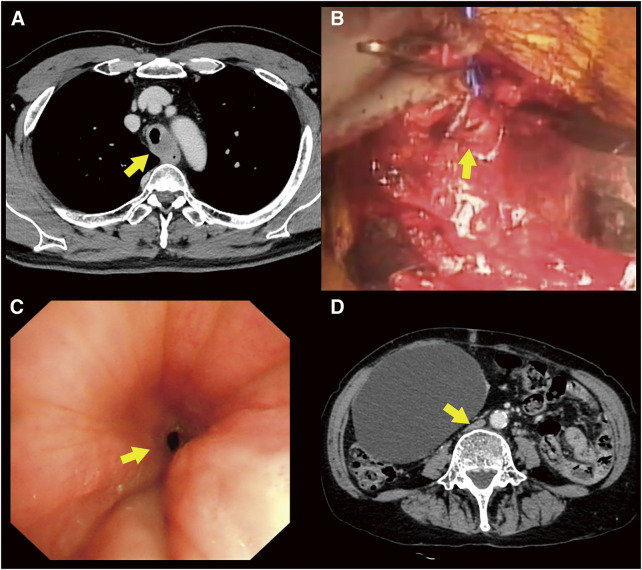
(A) Contrast-enhanced CT revealed a tumor 4.7 cm in length, extending cephalocaudally and dorsally to the trachea. A bronchoscopic biopsy confirmed an adenoid cystic carcinoma (arrow). (B) This intraoperative photograph of Case 1 shows that the dorsal half of the trachea has just been sutured (arrow) without the use of surgical field intubation. (C) Bronchoscopy in Case 2 revealed granulation stenosis (arrow) at the mouth side of the metallic stent inserted for tracheomalacia. (D) Contrast-enhanced CT in Case 2 showed compression of the inferior vena cava (arrow) by a large hepatic cyst. Because inserting a longer drainage cannula through the right femoral vein would be difficult, a drainage cannula was placed in the right internal jugular vein, and a return cannula was placed in the right femoral vein (VV-ECMO). CT, computed tomography; VV-ECMO, veno-venous-extracorporeal membrane oxygenation
